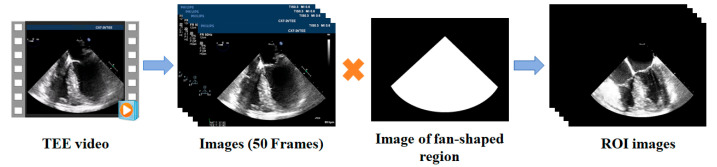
Generation of ROI images. TEE: transesophageal echocardiography; ROI: region of interest.
